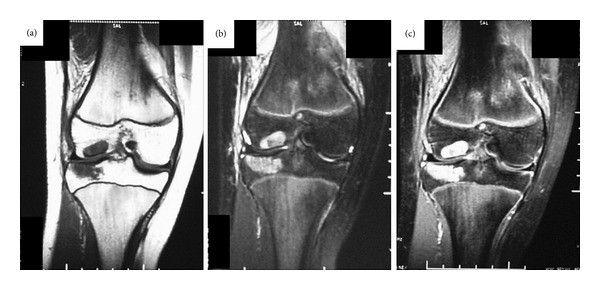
Magnetic resonance imaging (MRI) of the lesions in the distal epiphysis of the right femur and the proximal epiphysis of the right tibia. All lesions show a low signal intensity on a T1-weighted image (a) and a high signal intensity on a fat-suppressed T2-weighted image (b). The lesions were strongly enhanced by gadolinium ((c) T1-weighted images enhanced by gadolinium).
